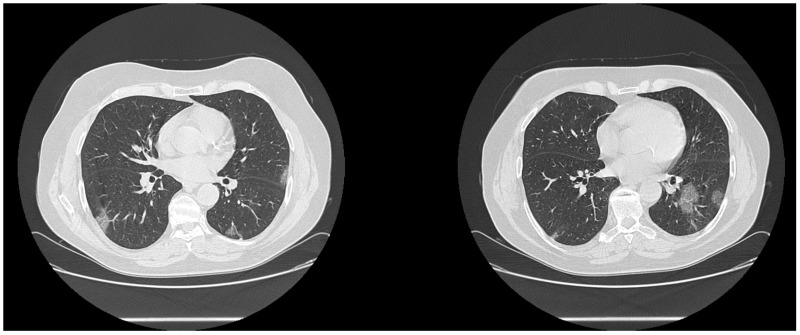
CT examination of a patient with clinical suspicion of COVID-19 infection and negative RT-PCR test. The CT images show the presence of GGO areas with typical roundish morphology and a predominantly peripheral distribution, a suspect picture for COVID-19 infection. The second RT-PCR test showed positive results.
